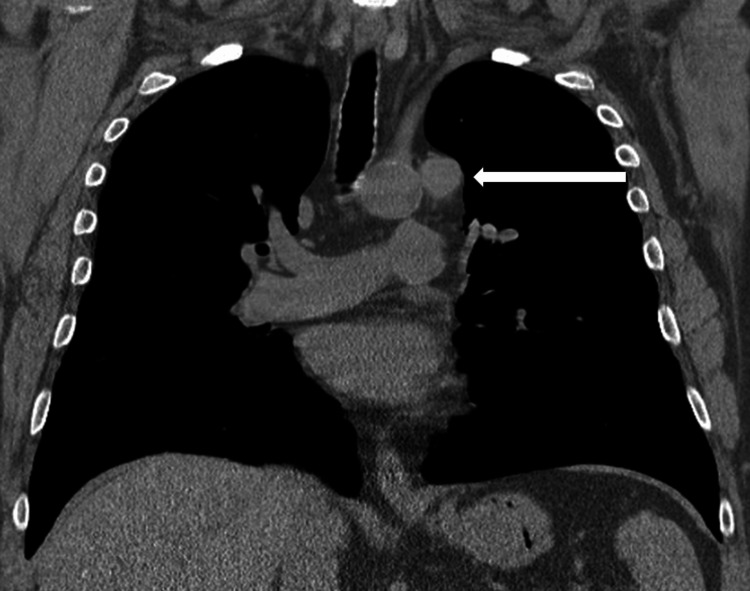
Coronal reformat of unenhanced chest CT imaging at the level of the trachea Coronal reformat of an unenhanced chest CT at the level of the trachea, showing a 2 cm lymphadenopathy located left to the aortic arch (arrow). One can also note the minor aortic arch calcifications.
